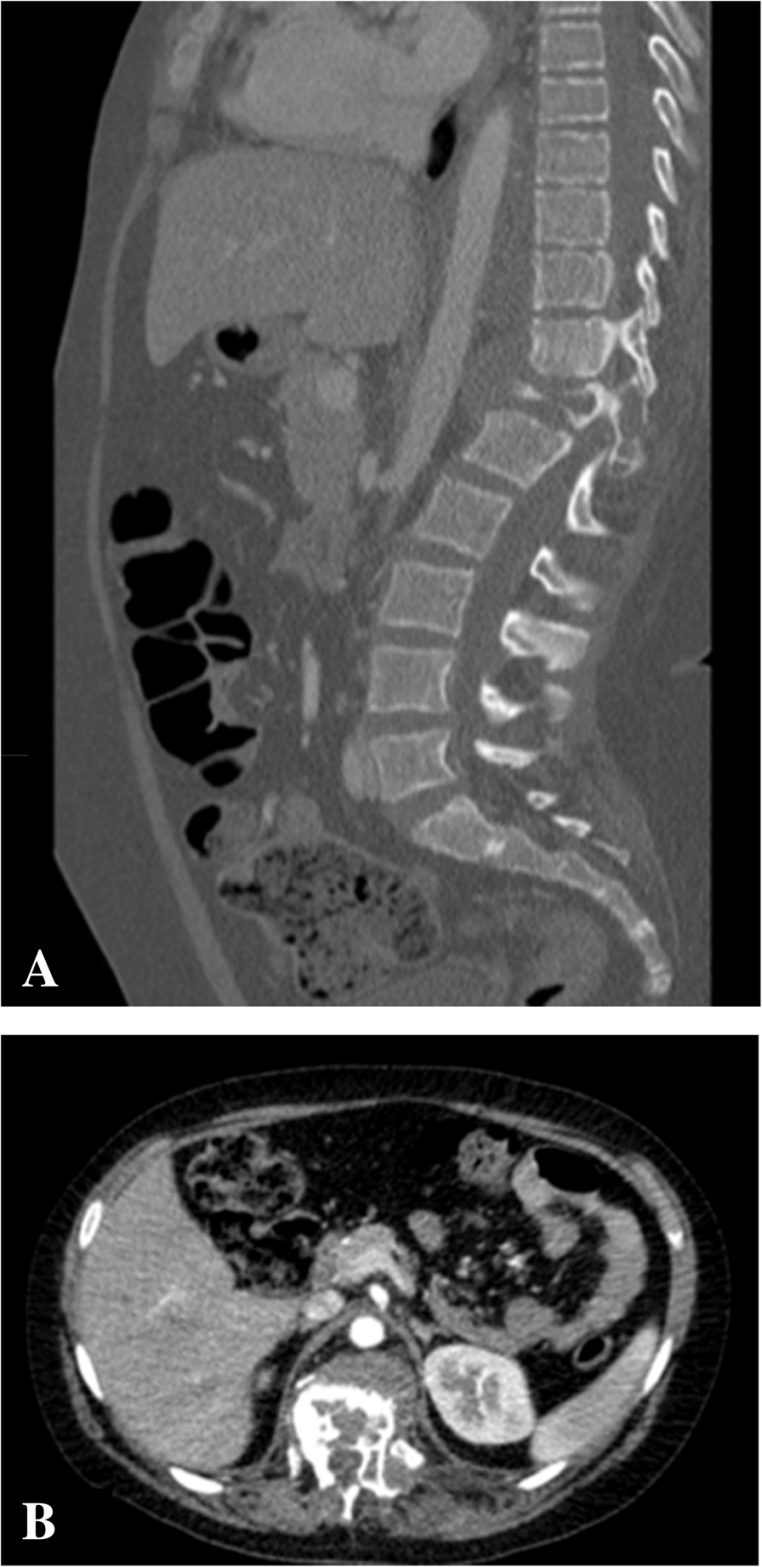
Initial computed tomography scan of chest and abdomen. Computed tomography scan of chest and abdomen, contrast-enhanced multiplanar reformatted (MPR) images in the sagittal (A) and axial (B) plans, showing a paravertebral mass extended up to Th8 level and to L3 level leading to vertebral collapse at Th12, thinning of the spinal canal and cord compression, and expanding toward retroperitoneal structures
